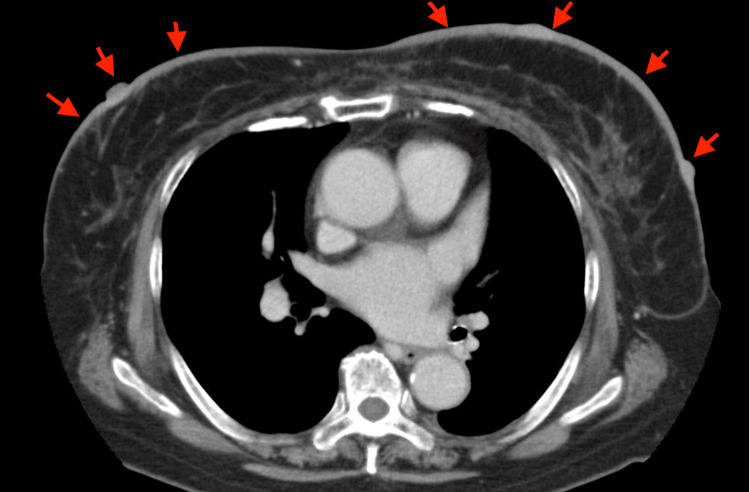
Chest CT imaging. Chest CT imaging shows bilateral breast skin thickness and multiple nodules (arrows). No distant metastasis is observed.
